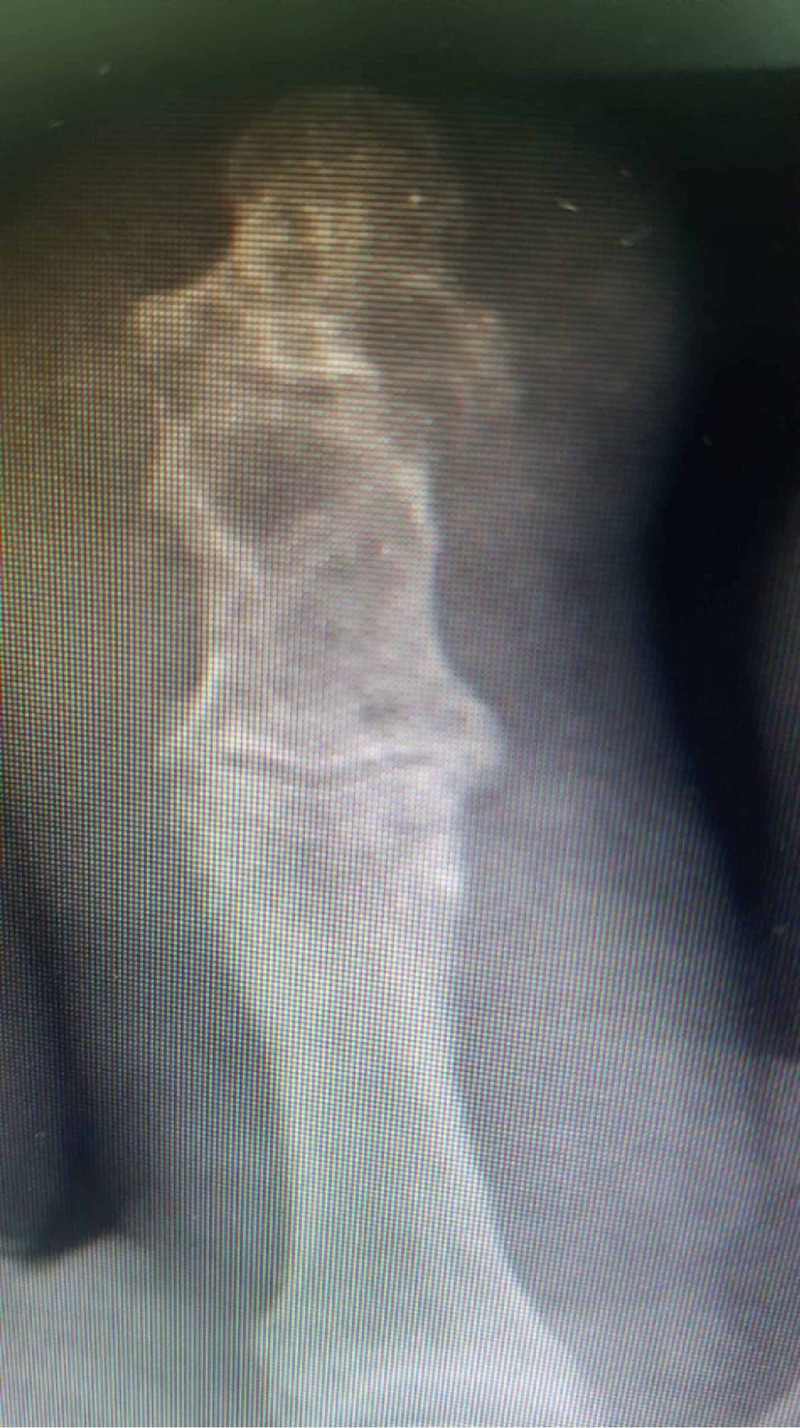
X-ray of the second toe on initial presentation showing no signs of osteomyelitis or bony destruction
